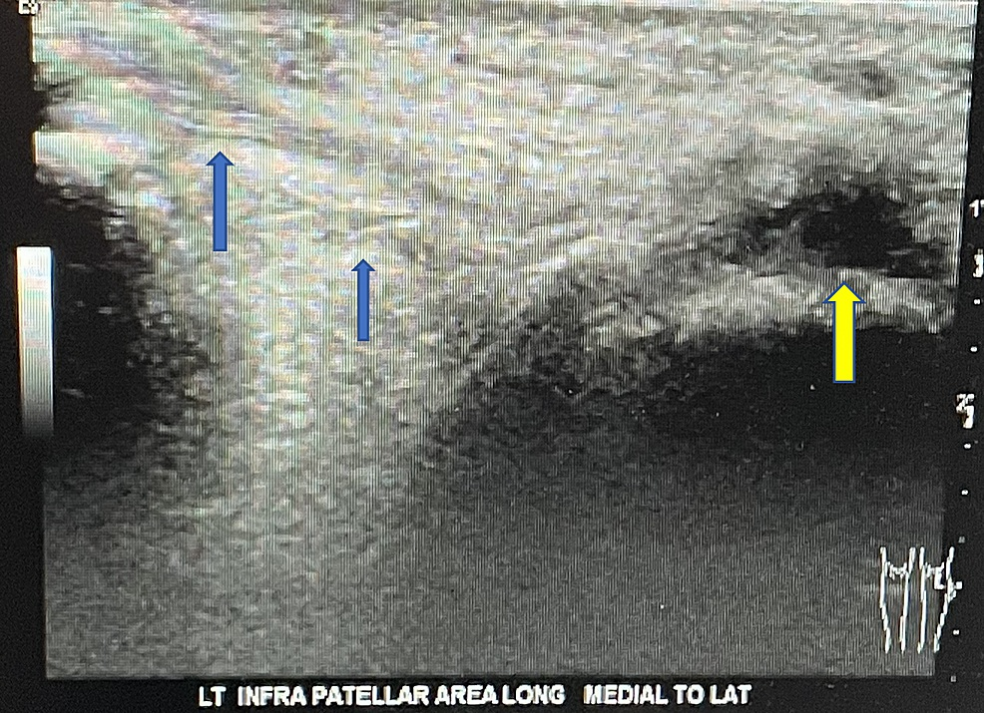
Ultrasound of the left knee showing patellar tendon rupture. No normal-appearing patellar tendon is visualized within its expected location. The attachment at the inferior pole of the patella appears attenuated, with fibers seen extending anterior to the patella (blue arrows). The tibial tuberosity is indicated by the yellow arrow.
